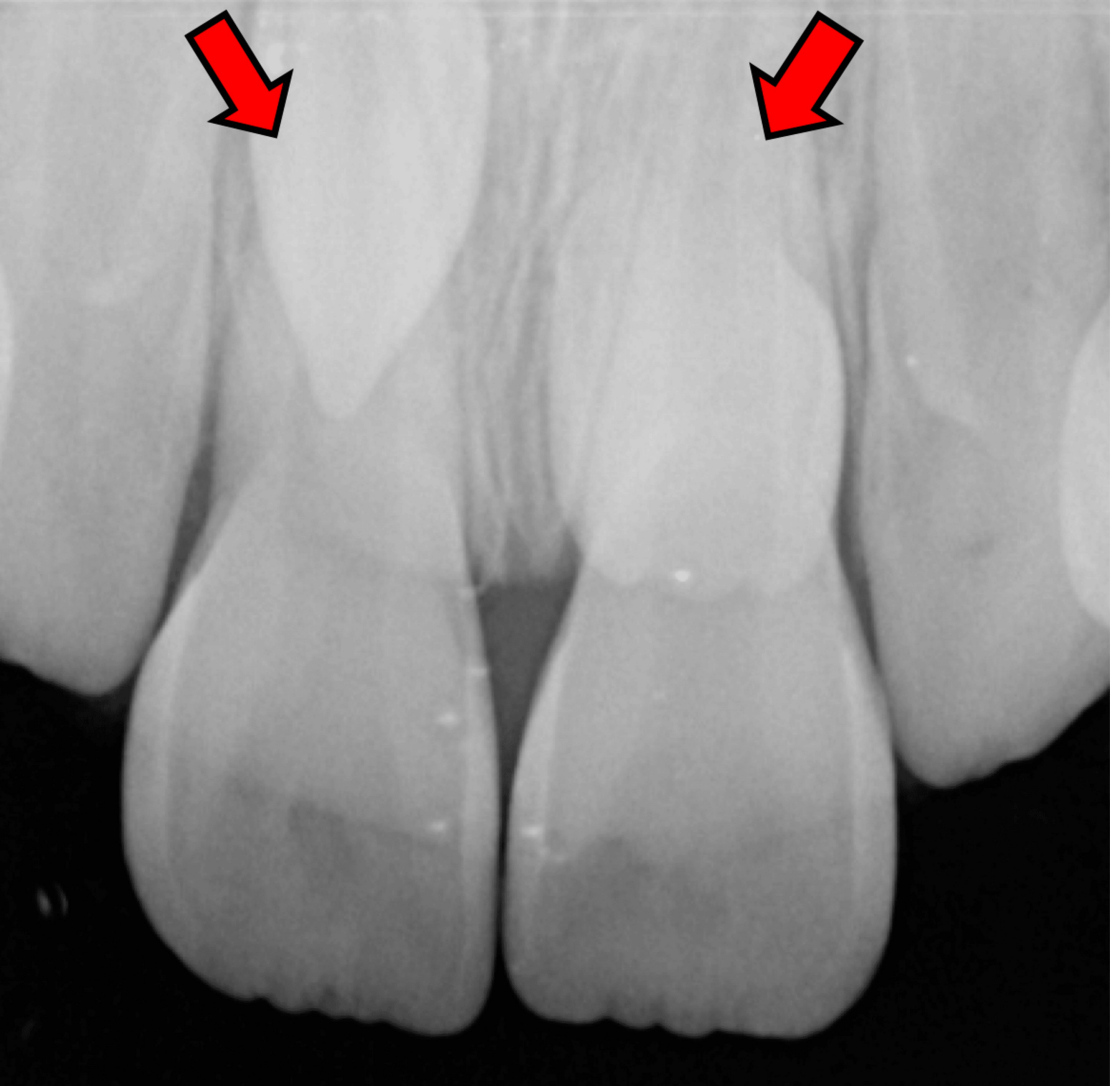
Periapical radiograph The red arrows point to the impacted supernumerary teeth, revealing their orientation and proximity to the roots of the permanent maxillary central incisors.
